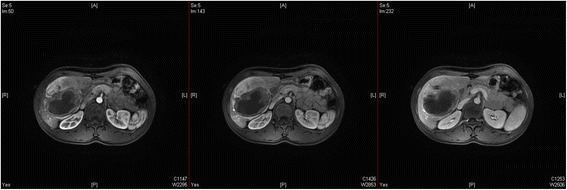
Magnetic resonance imaging scan of the upper abdomen. The mass exhibits uneven strengthening and the internal liquefied necrotic area has no strength
